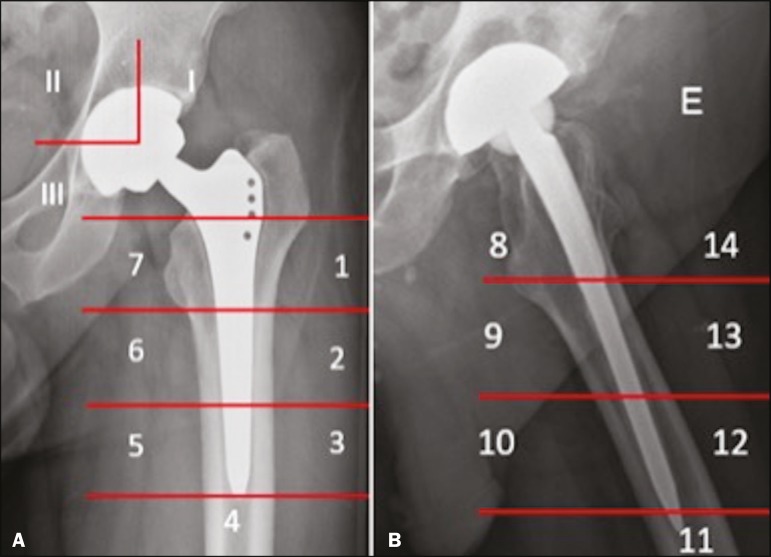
Femoral and acetabular zones in the evaluation of osteolysis. A: Anteroposterior X-ray showing the first seven femoral zones and the three acetabular zones. B: Lateral X-ray showing the seven additional femoral zones.
